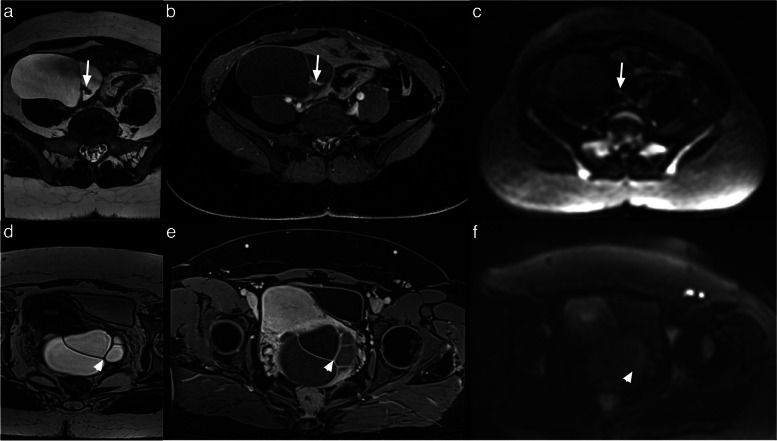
MRI appearance of a thick (first row, white arrow) and a thin septa (second row). a Axial T2-weighted sequence showing a thickened septa with tissular component. b Axial late-gadolinium T1-weighted sequence showing a tissular enhancement. c Axial DWI abdominal and pelvic scan (*b*: 1200 s/mm^2^) with hypersignal of the tissular component. d Axial T2-weighted sequence showing a thin septa. e Axial late-gadolinium T1-weighted sequence showing a thin enhancement. f Axial DWI abdominal and pelvic scan (*b*: 1200 s/mm^2^) showing no hypersignal
